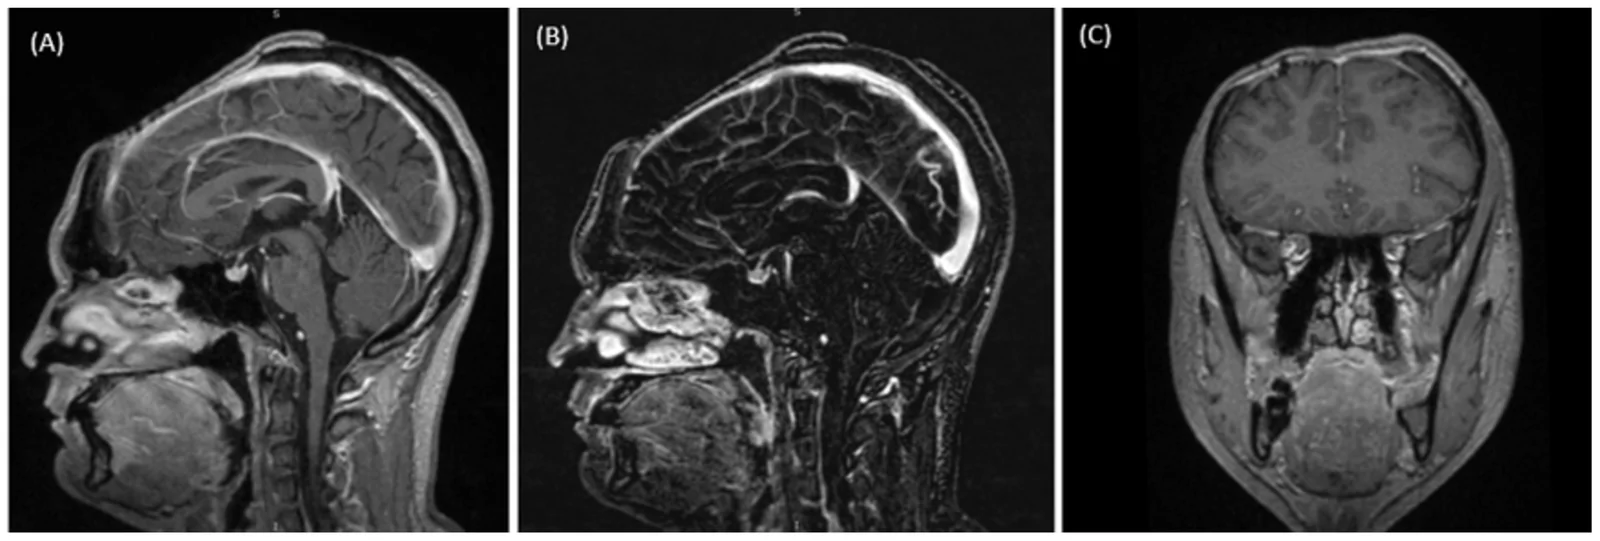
Follow-up contrast-enhanced brain MRI. Sagittal postcontrast 3D T1-weighted image (A), sagittal subtraction image (B), and coronal reformatted postcontrast image (C) demonstrate complete radiological resolution of the previously identified frontal lesion, with no residual or recurrent contrast-enhancing tumoral tissue.
